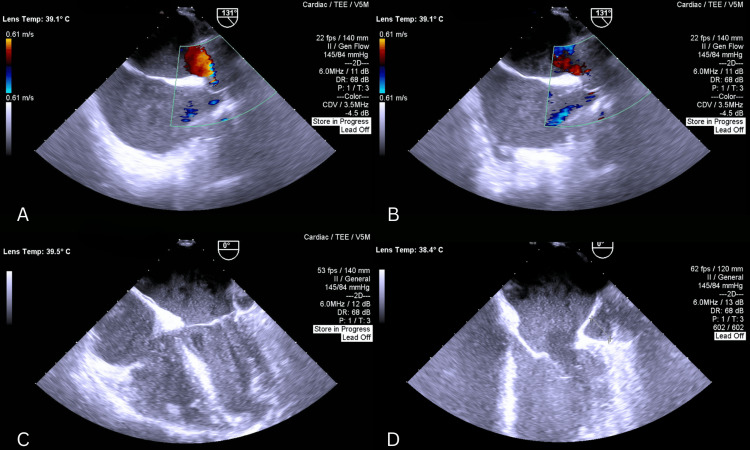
(A, B) Bicaval views with Doppler demonstrating closed ASD with no residual flow across the septum. (C) Four-chamber view showing a reduced right ventricular size and a dilated coronary sinus. (D) Measurement of dilated coronary sinus diameter ASD: Atrial septal defect
